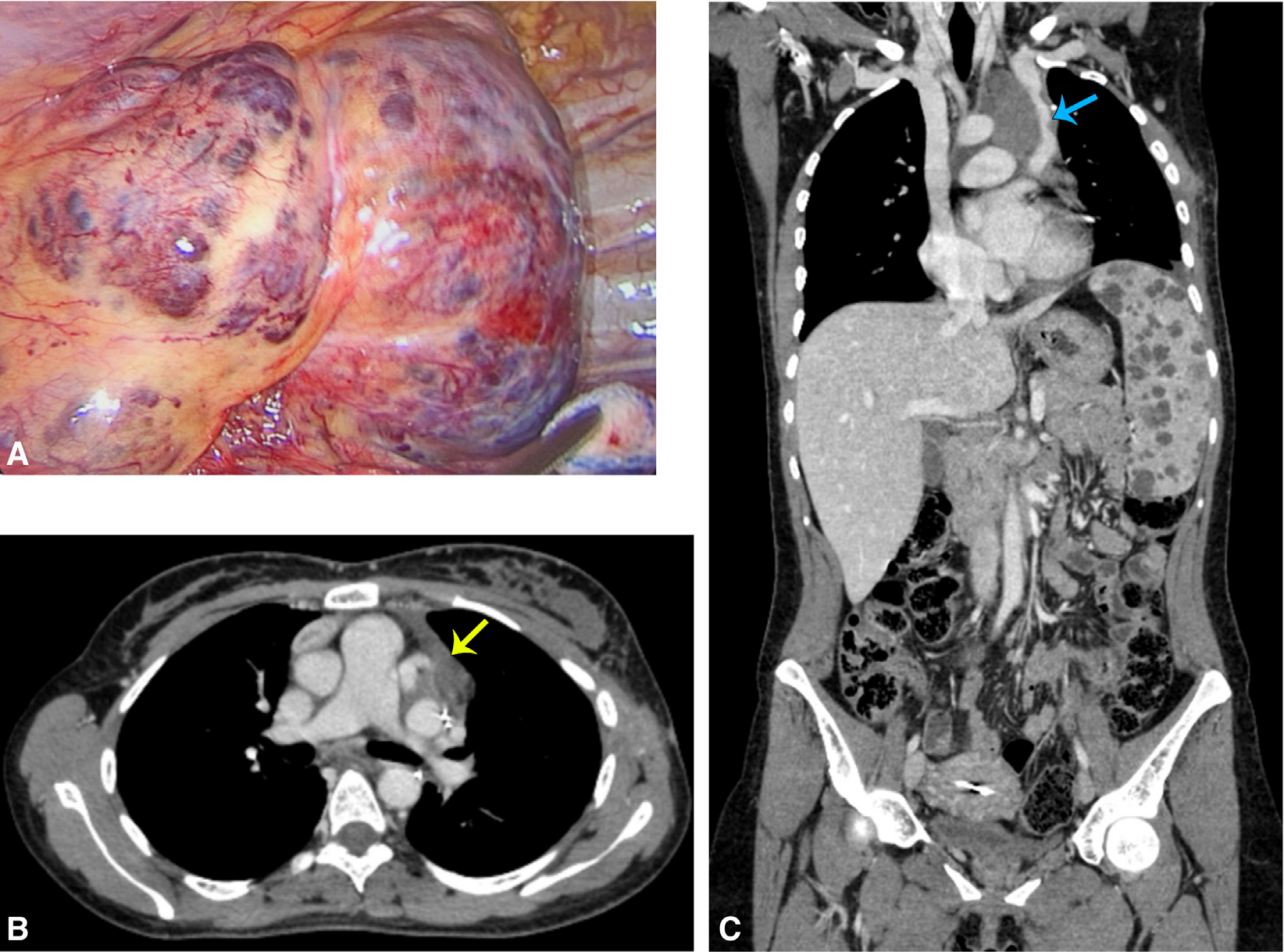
A, Intraoperative image showing the vascular surface of the lesion. B, Venous phase of postoperative computed tomography scan showing the remnants of the resected lesion (*yellow arrow*). C, Sagittal view showing the left superior vena cava postoperatively (*blue arrow*).
